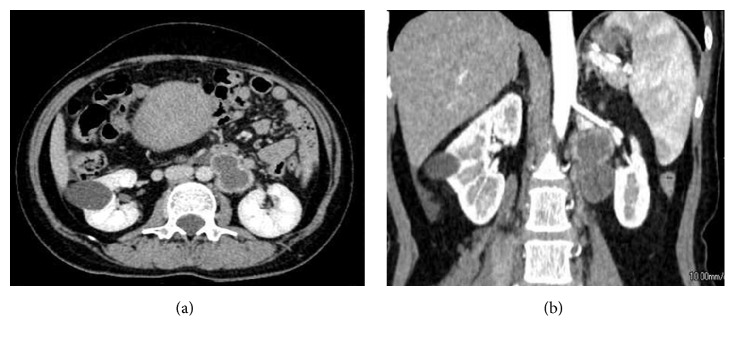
Computed tomography scan showing that the bilocular cystic mass is located caudally from the left renal hilus without solid parts.
